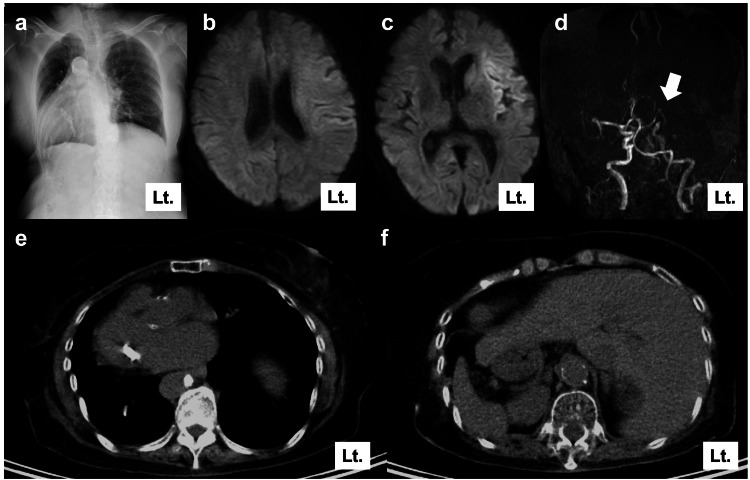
Pre-procedural findings a: Chest X-ray showing a dextrocardia. b, c: Magnetic resonance imaging showing a hyperintense area in the left insular cortex and left frontal operculum. d: Magnetic resonance angiography imaging showing left distal internal carotid artery occlusion (white arrow). e, f: Chest computed tomography showing complete situs inversus totalis obtained during breast cancer treatment
